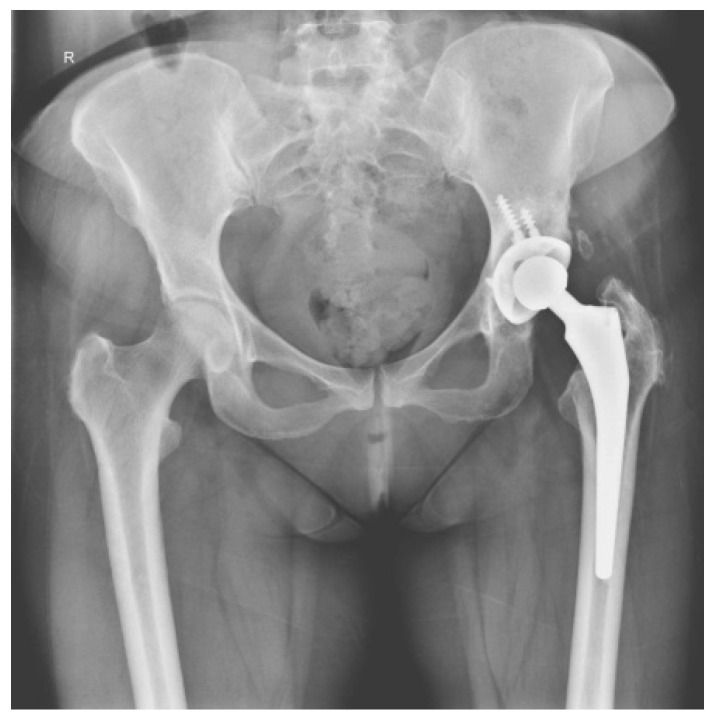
Pelvis X-ray with both joints—left total hip arthroplasty with augmented total non-cemented prosthesis with screws in the acetabulum—preoperative aspect.
